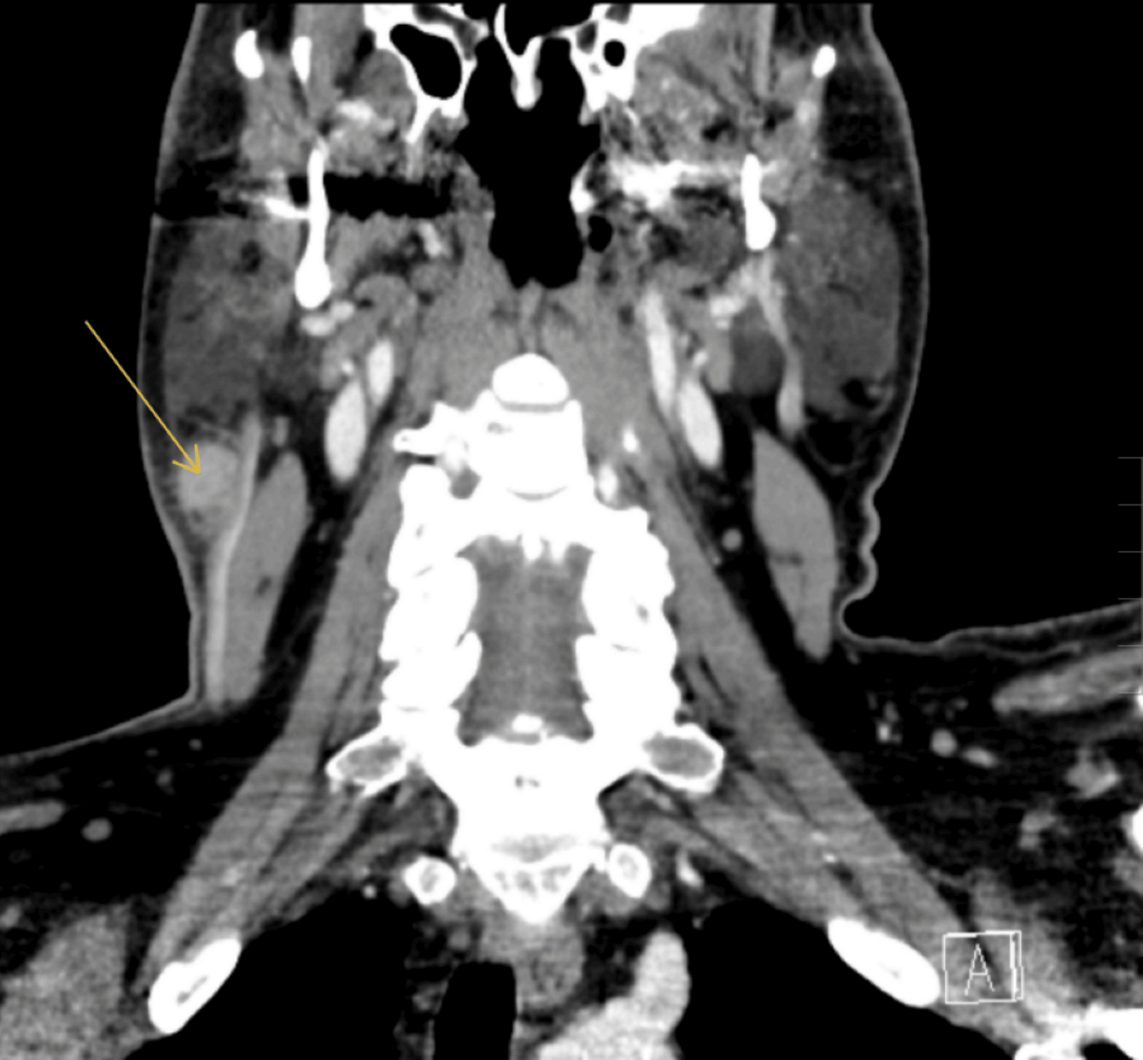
Coronal CT denoting prominent cluster of cervical lymph node enlargement
